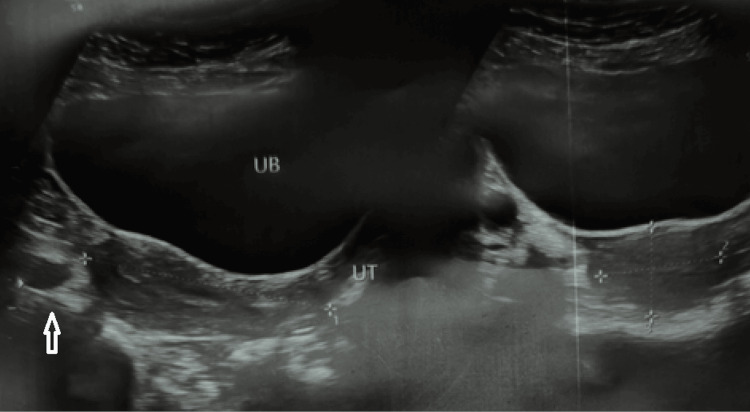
Ultrasonography of the abdomen and pelvis revealing normal uterine and adnexal anatomy, with no evidence of outflow obstruction
